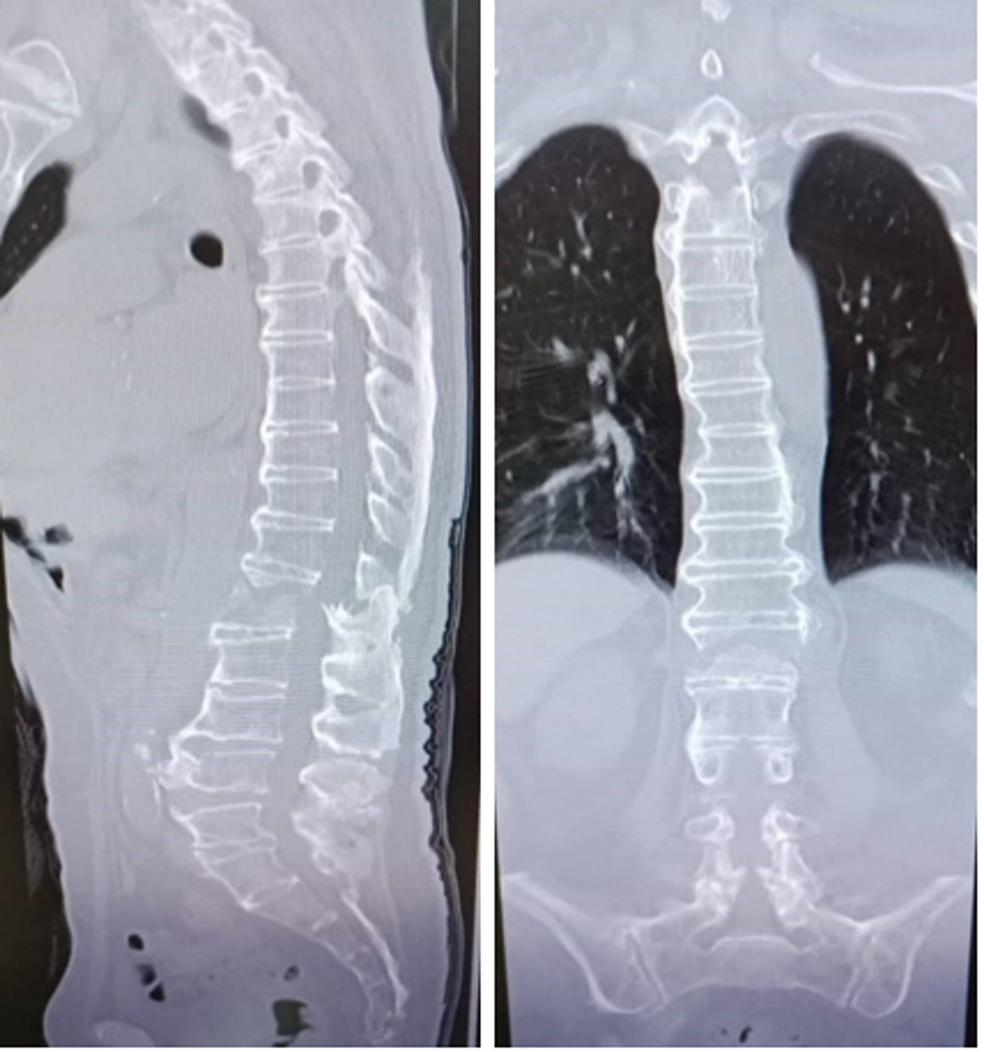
CT scan showing the L1 Chance fracture
